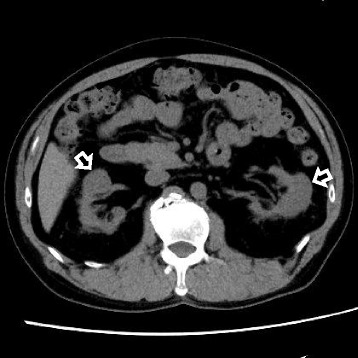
Findings of computed tomography after treatment. Focal atrophy of kidneys. Mass-like regions before treatment did not show atrophic change by computed tomography
